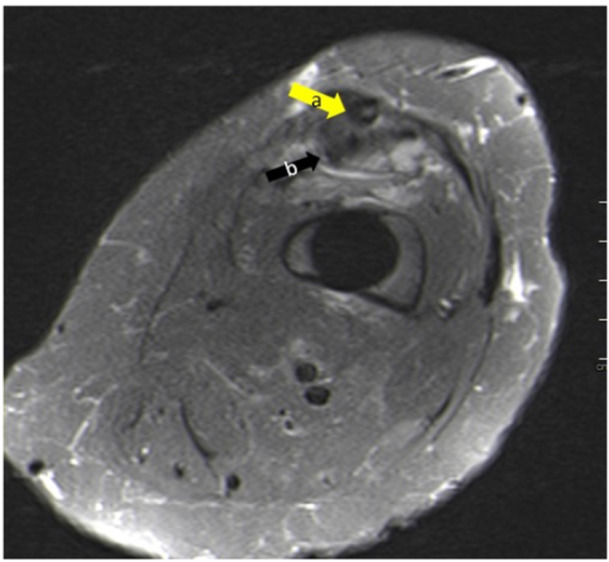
Axial MRI image showing the (a) graft and (b) the intact fibres of the quadriceps. MRI, magnetic resonance imaging.
